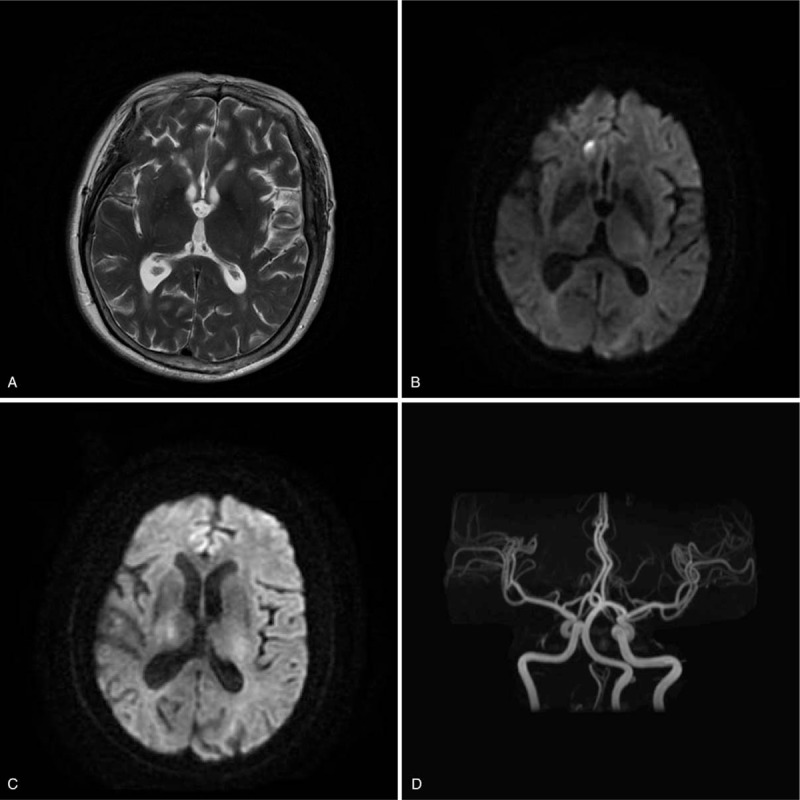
Head MRI performed on September 29th. (A) Patched long T2 signal in the right frontal lobe; (B) high DWI signal in the right frontal lobe; (C) suspicious high DWI signal in bilateral frontal-parietal lobes; (D) Head MRA, suggesting atherosclerotic changes. DWI = diffusion-weighted imaging, MRI = magnetic resonance imaging.
